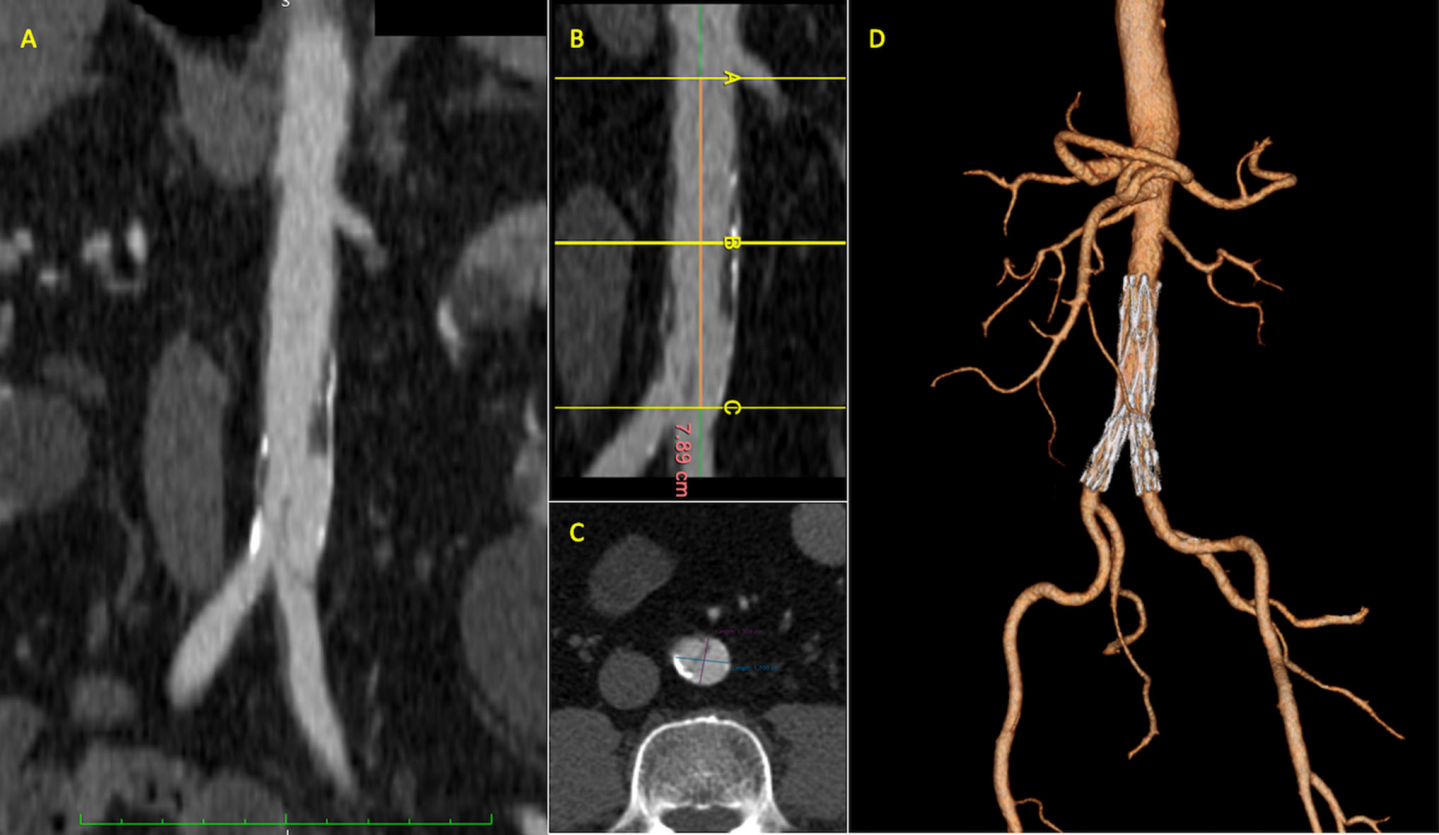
Preoperative CTA with maximum intensity projection (MIP) showing an infrarenal aortic dissection, involving of the origin of the left common iliac artery (a); centerline showing a limited length of the infrarenal aorta (less than 8 cm) (b); axial projection showing the dissection flap in correspondence of the distal abdominal aorta with limited aortic diameter (approx. 17 × 15 mm) (c); postoperative 24-month follow-up CTA 3D reconstruction showing the exclusion of dissection with patent true lumen and disappearance of the false lumen (d)
